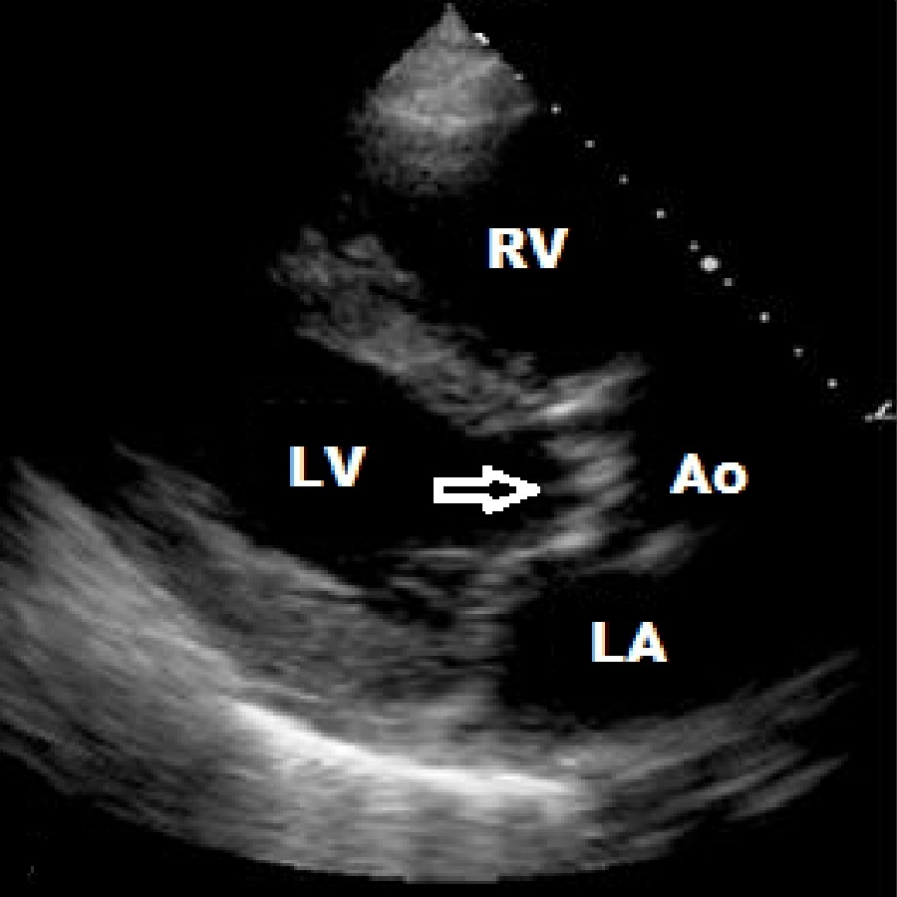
Transthoracic echocardiogram (parasternal long axis view) showing severe aortic stenosis (arrow) RV - right ventricle, LV - left ventricle, Ao - aorta, LA - left atrium.
